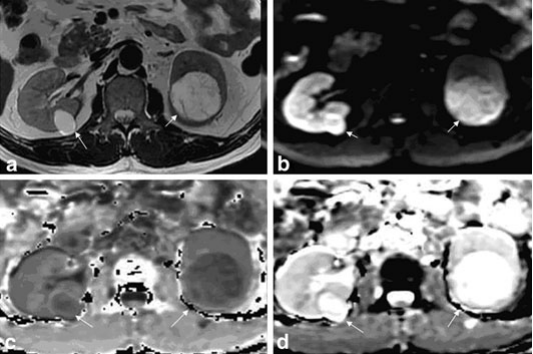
Kidney cyst in a 52 years old woman. a T2WI showed two cysts (*arrow*) in left and right kidney, respectively. Visible thin line separator can be seen in the *left* kidney cyst. b DWI showed high signal intensity. c EADC showed low signal intensity. The separator within the *left* kidney lesions showed slightly higher signal intensity. d ADC showed significantly high signal intensity. Part of the structure within the *left* kidney lesions was covered
